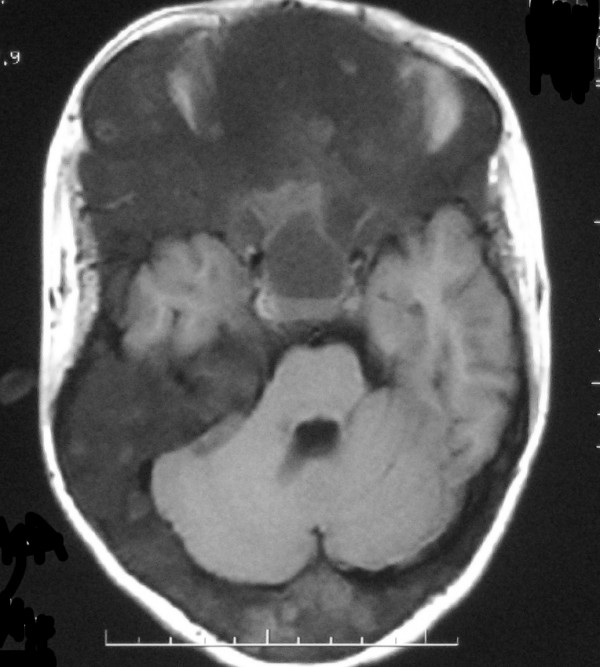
Axial T1-weighted MR images show abnormal thickening of the bone involving the frontal bone, sphenoid bone, lateral and medial orbital walls, with right canal is shallow and optic nerve is compressed.
